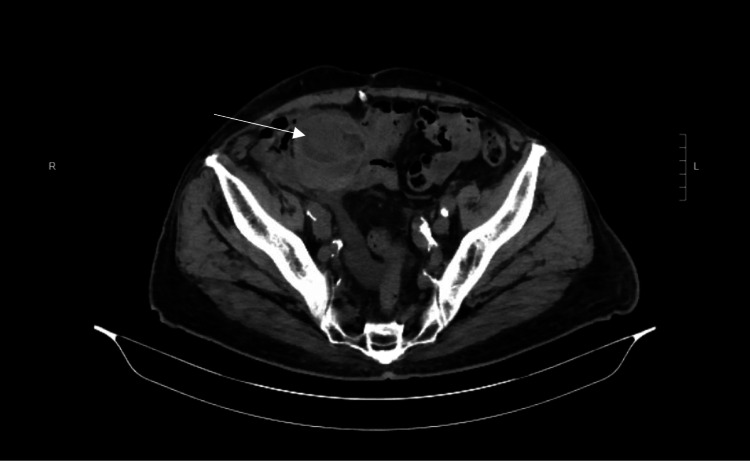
Postoperative axial CT scan of a 78-year-old male patient, with ascites from end-stage renal disease on peritoneal dialysis and a right-sided indirect inguinal hernia, following the described inguinal hernia repair. The arrow indicates the retroperitoneal testicle.
